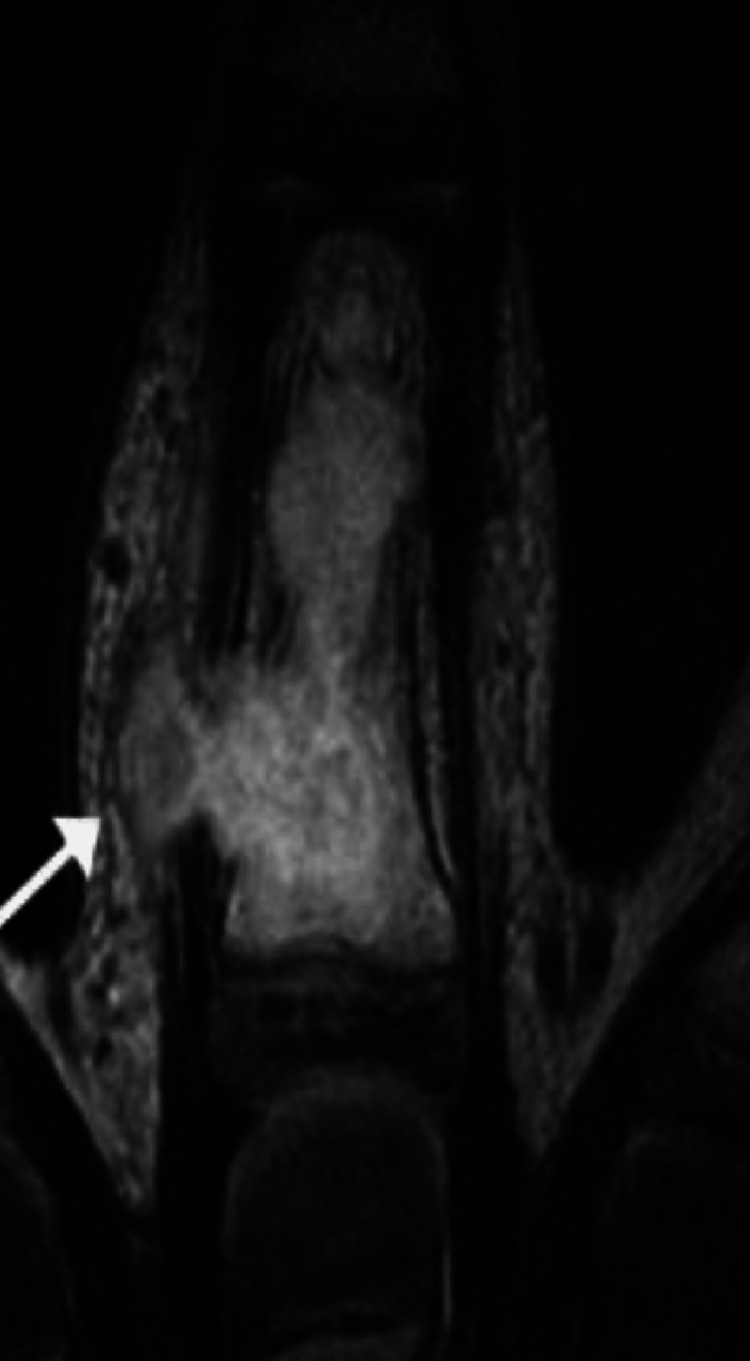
A 17-month-old girl diagnosed with BCG osteomyelitis of the phalanx The coronal T2-weighted image of the right hand shows the abscess extending into the subcutaneous tissue disrupting the cortex [17].
